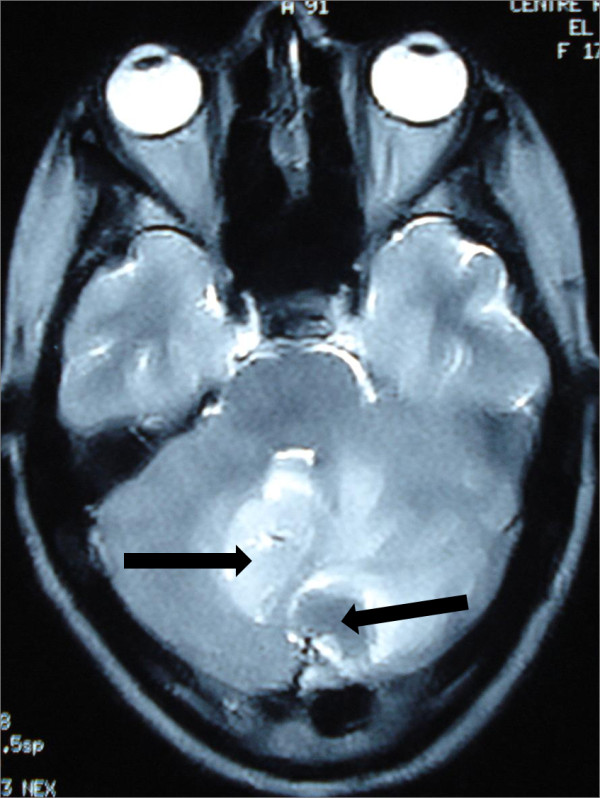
The processes are heterogeneous on a T2-weighted image (arrow) with discrete surrounding edema. The cerebellar lesion measures 3×4cm in size.
